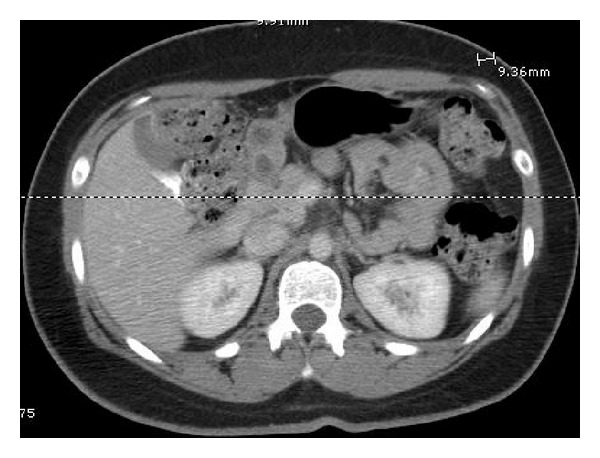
CT scan demonstrated four cystic lesions in the medial wall of distal antrum and pylorus measuring approximately one cm each, suggestive of duplication cysts.
